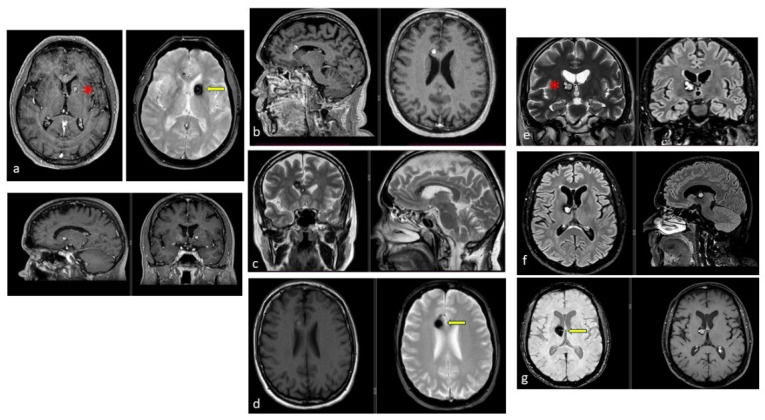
Examples of deep-seated asymptomatic CMs. (a). Axial, sagittal, and coronal T1-weighted MRI with gadolinium and axial GRE T2*-weighted MRI of the left caudate nuclei CM. (b). Sagittal and axial T1-weighted MRI with gadolinium. (c). Coronal and axial GRE T2*-weighted MRI and (d). Axial T1 and GRE T2*-weighted MRI, showing a CM of the corpus callosum. (e). Coronal T2-weighted and FLAIR MRI. (f) Axial and sagittal FLAIR MRI and (g). Axial GRE T2* and T1-weighted MRI showing a right thalamic CM. Note the “blooming artifact” on axial GRE T2*-weighted MRI due to pools of deoxygenated blood and deposits of hemosiderin and calcium (yellow arrows) and the classic “popcorn” appearance on FLAIR-weighted MRI with mixed signal intensity, expression of various blood breakdown products (red asterisk) of the CMs.
